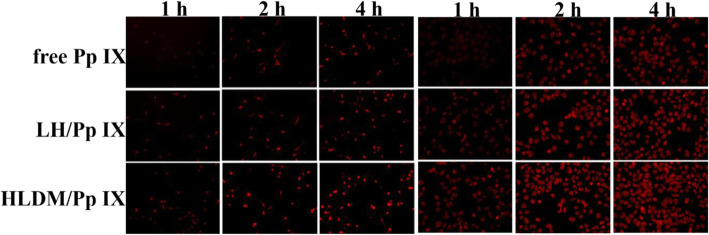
Uptake of free Pp IX, Pp IX-loaded LH, and Pp IX-loaded HLDM micelles in CT-26 (left) and A549 (right)
